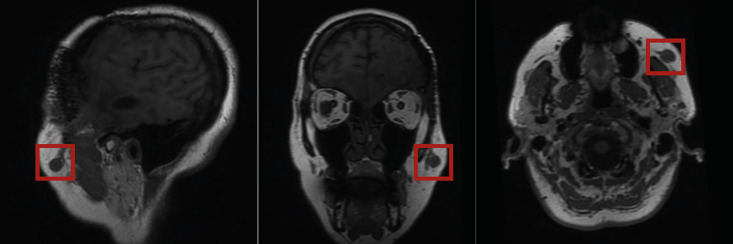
Sagittal, coronal, and axial reconstruction planes in T1-weighted sequences. A well-circumscribed round lesion measuring approximately 2 cm arises from the soft tissues, is isointense to gray matter, and shows a tail-like extension toward the underlying musculature.
